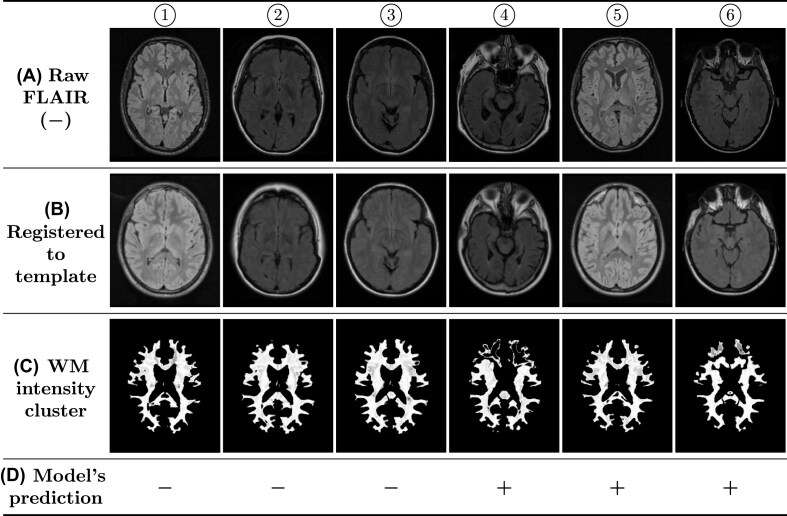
Example of 6 MRIs (① to ⑥) *without* WM abnormality. (A) Raw FLAIR images, (B) the MRI 3 times registered to the MNI template (the middle slice shown), (C) the obtained intensity clusters (thresholded) used for testing the model, and (D) the prediction of the model in setting *A*00 for the presence of WM abnormalities ($\boldsymbol {+}$: with WM abnormality, $\boldsymbol {-}$: without WM abnormality). This figure shows 3 cases of true negative and 3 cases of false positive. The WM intensity cluster slice corresponds to the registered MRI slice, but not exactly to the raw MRI slice, due to deformations from nonlinear registrations. MRI source: ①, ⑤ [83]; ②, ③, ④ [81]; and ⑥ [80].
